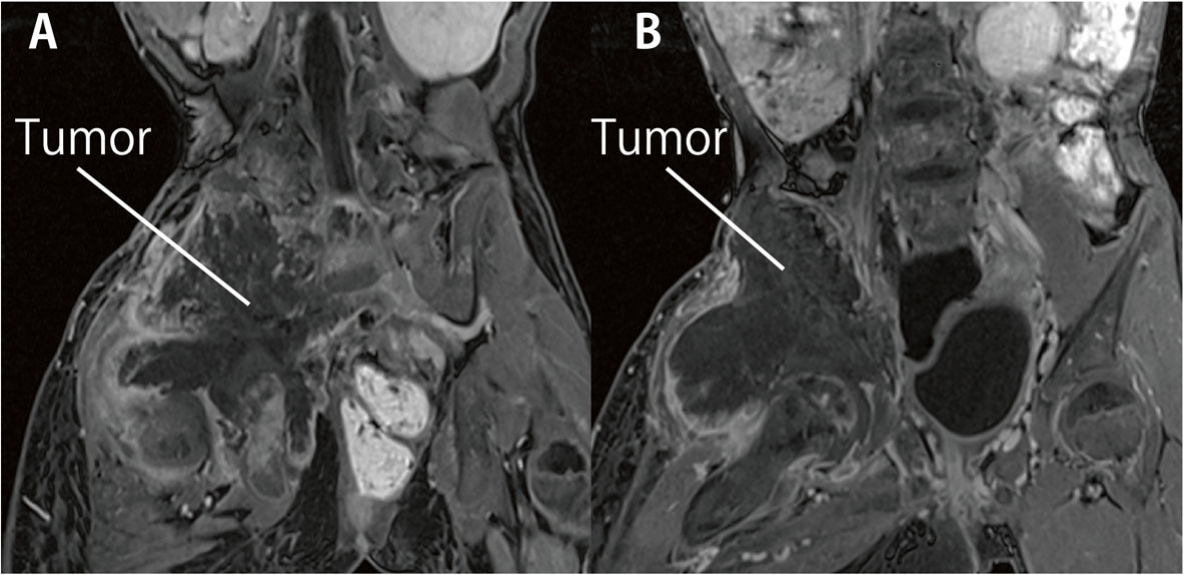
Magnetic resonance imaging of the tumor. Magnetic resonance imaging shows that the iliac tumor had invaded the right lumbar plexus. Slightly different sections are shown (a and b)
